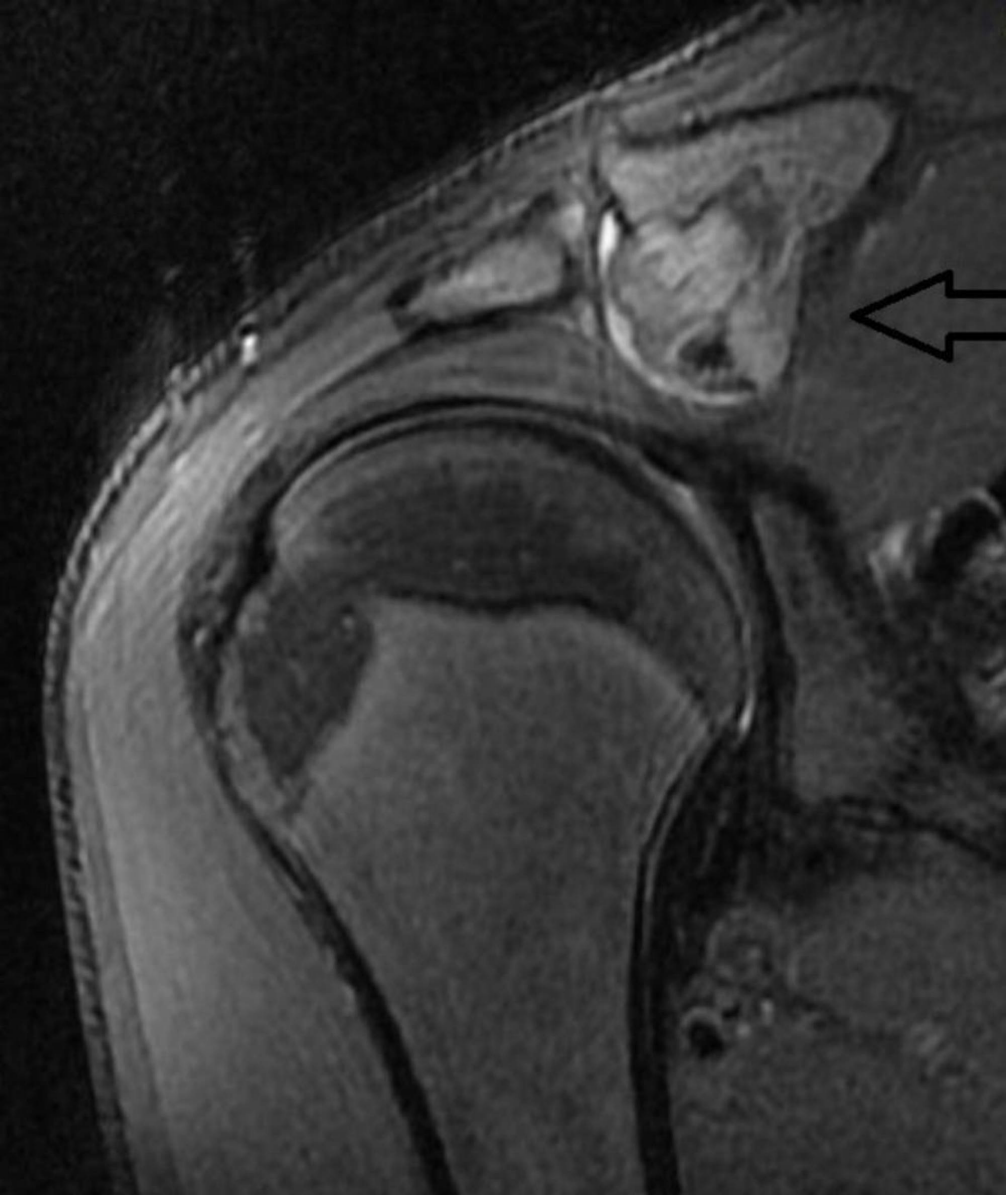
Magnetic resonance imaging (MRI) of the lesion highlights benign characteristics.
